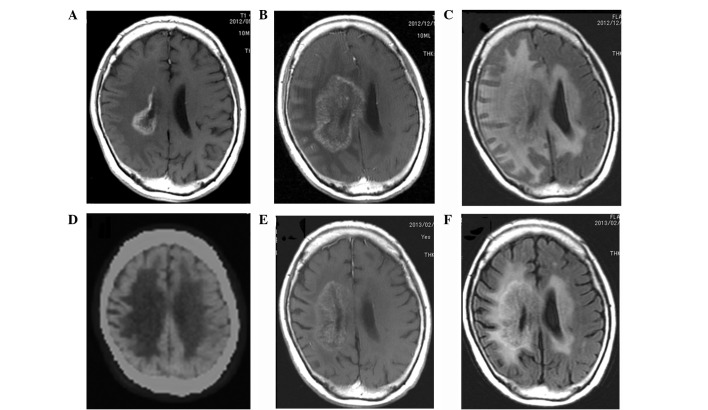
Imaging studies of the patient’s radiation necrosis. (A) Gadolinium-enhanced MRI showed an enhanced lesion caudal to the original lesion that appeared approximately two years following the initial surgery. (B) Gadolinium-enhanced MRI and (C) FLAIR showed growth in the size of the lesion following the second round of CyberKnife radiotherapy. (D) ^11^C-methionine positron emission tomography showed that the lesion and its surrounding area exhibited no hot spots, which is consistent with the diagnosis of radiation necrosis rather than tumor recurrence. Bevacizumab treatment showed a marked effect on the radiation necrosis lesion as visualized by gadolinium-enhanced (E) MRI and (F) FLAIR. MRI, magnetic resonance imaging; FLAIR, fluid attenuated inversion recovery.
